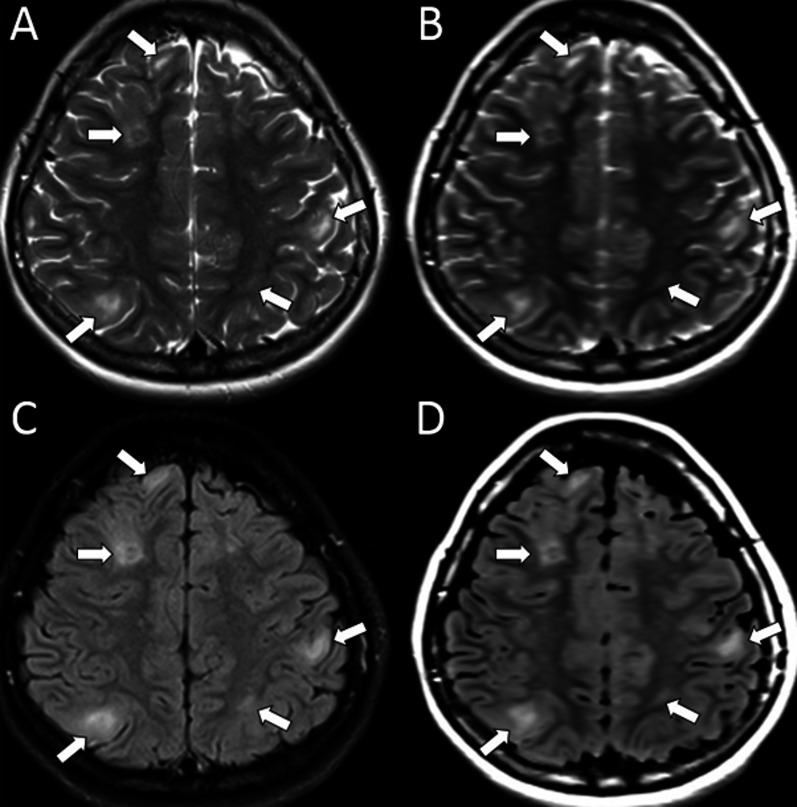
Synthetic (Sy) T2-weighted (B) and Sy-FLAIR (D) images show cortical/subcortical tubers accurately as on the conventional T2W (A) and FLAIR (C) images
